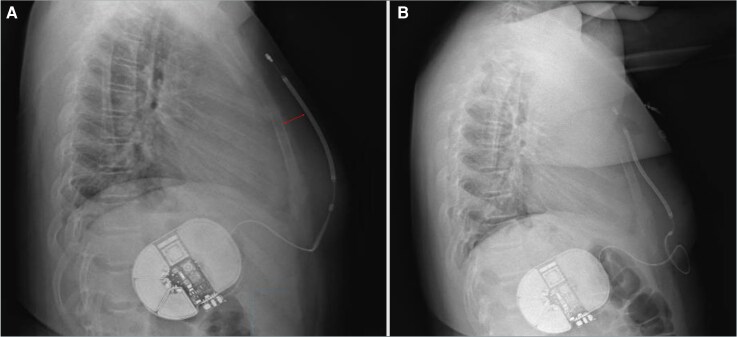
Pre- and post-intervention chest radiographs. (*A*) Increased distance between the electrode and the sternum (arrow) due to subcutaneous fat accumulation, resulting in elevated shock impedance and ineffective therapies. (*B*) Proper electrode positioning after surgical repositioning.
